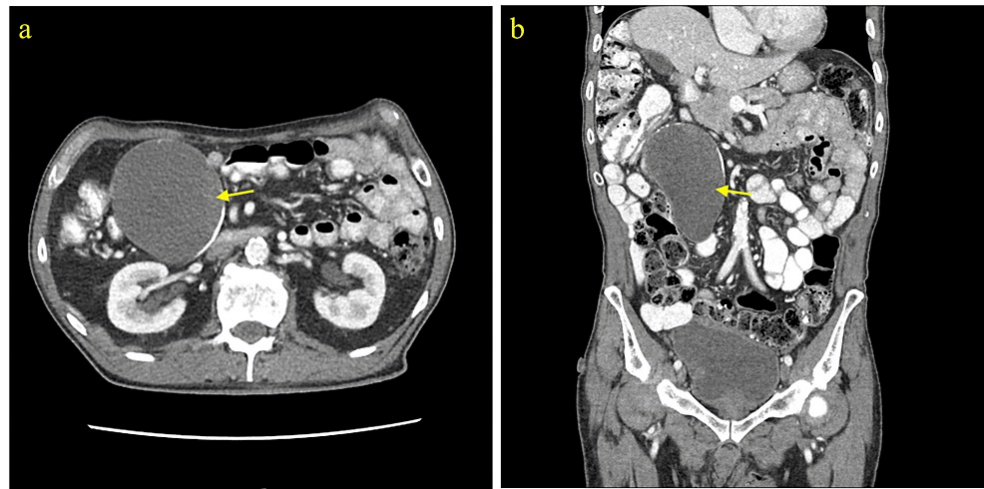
CT abdomen and pelvis with contrast (Case 2). a) Axial view and b) coronal view showing a cystic lesion with peripheral calcification in the right abdomen. Subsequent exploratory laparotomy and appendectomy revealed a large cystic appendiceal tumor. Pathology of the lesion was consistent with LAMN. LAMN, low-grade appendiceal mucinous neoplasm
